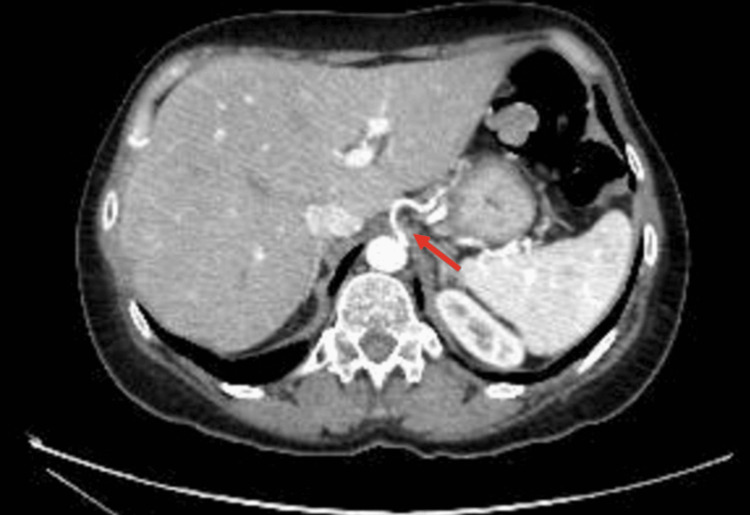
Axial CT demonstrating stenosis of the left gastric artery as it arises from the aorta The red arrow denotes the left gastric artery, slightly narrow at its origin, from the abdominal aorta
